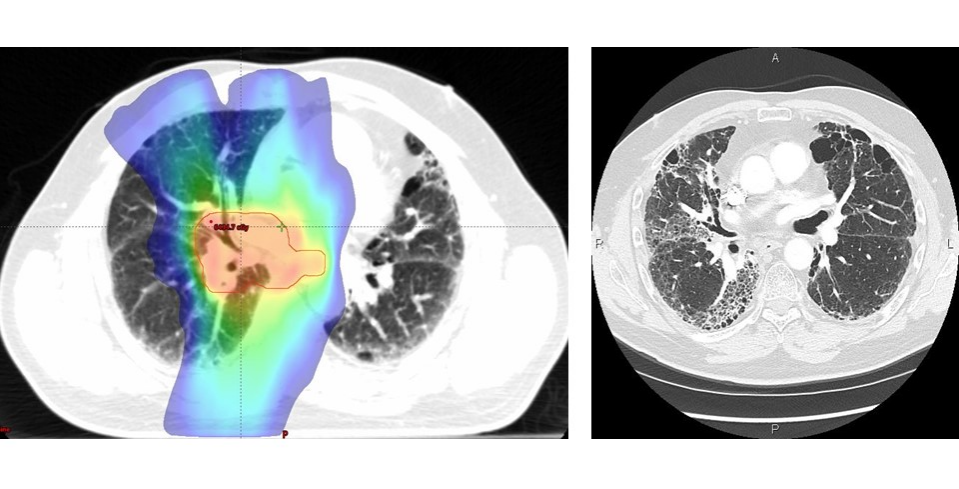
Color wash of the dose distribution on a radiation therapy planning computed tomography for a patient with lung cancer (left). The blue edge represents the 20 gray dose line, which is the recognized dose associated with increased risk of radiation pneumonitis. The same patient’s 3-month follow-up computed tomography image showed opacity indicating a partial filling of the air spaces in the lungs. These radiologic changes are representative of radiation pneumonitis in the radiation field (right).
